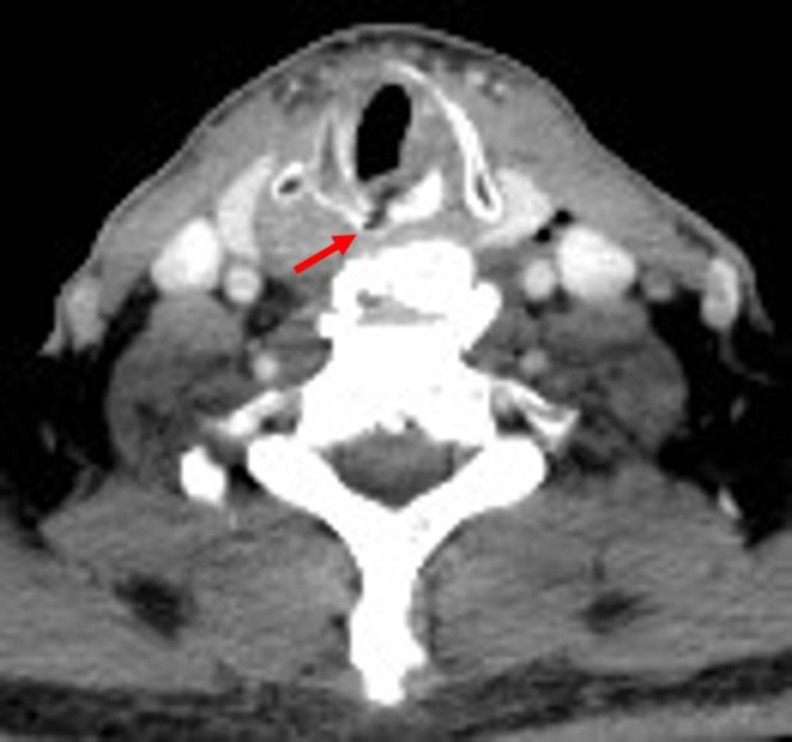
Neck CT (axial scan) showing the fracture of the cricoid plate (red arrow)
